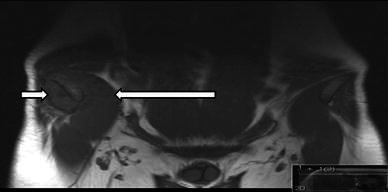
Coronal T1-weighted image. Note the slightly hyperintense haematoma (*short white arrow*). Signal is compared to skeletal muscle (*long white arrow*)
